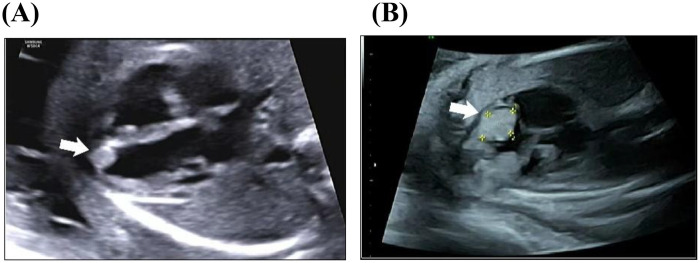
Prenatal ultrasound images of fetal cardiac rhabdomyomas (CR) in Two cases. (A Case 1: White arrow indicates multiple hyperechoic CR nodules in the fetal bilateral ventricles. (B) Case 2: White arrow indicates multiple hyperechoic CR nodules in the fetal bilateral ventricles, with the larger one (1.3 × 1.0 × 1.2 cm) in the right ventricle.
